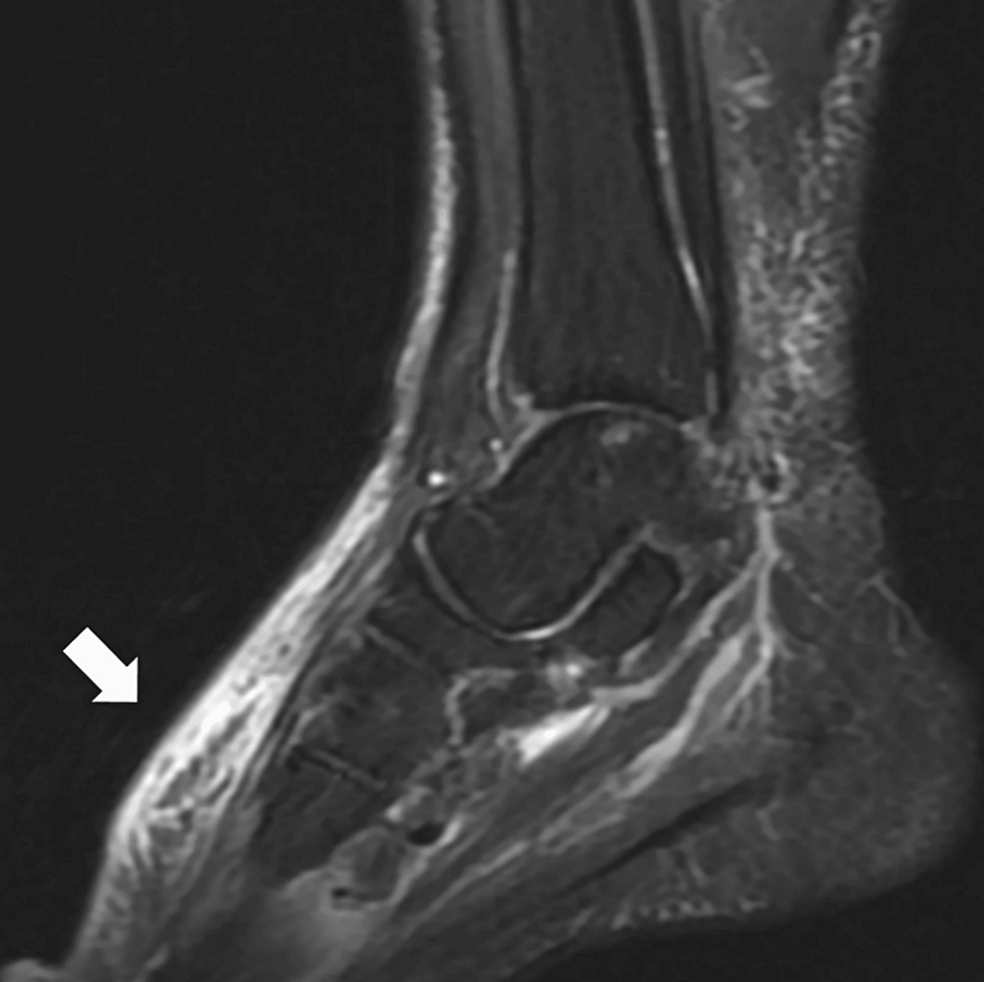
Right foot magnetic resonance imaging showing high signal range in the soft tissue of the right dorsal foot (white arrow)
